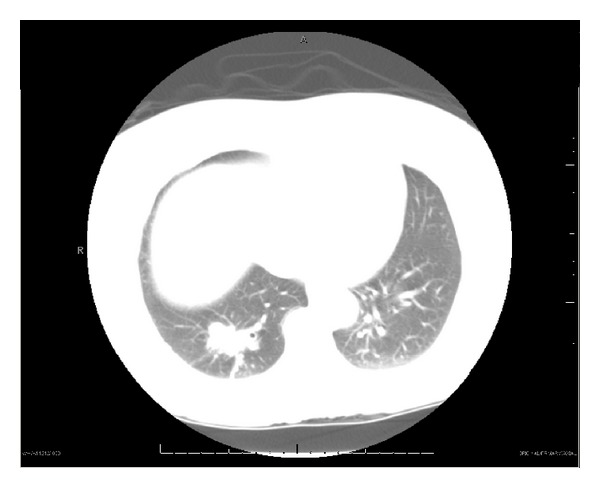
CT chest: right lower lobe spiculated mass.
